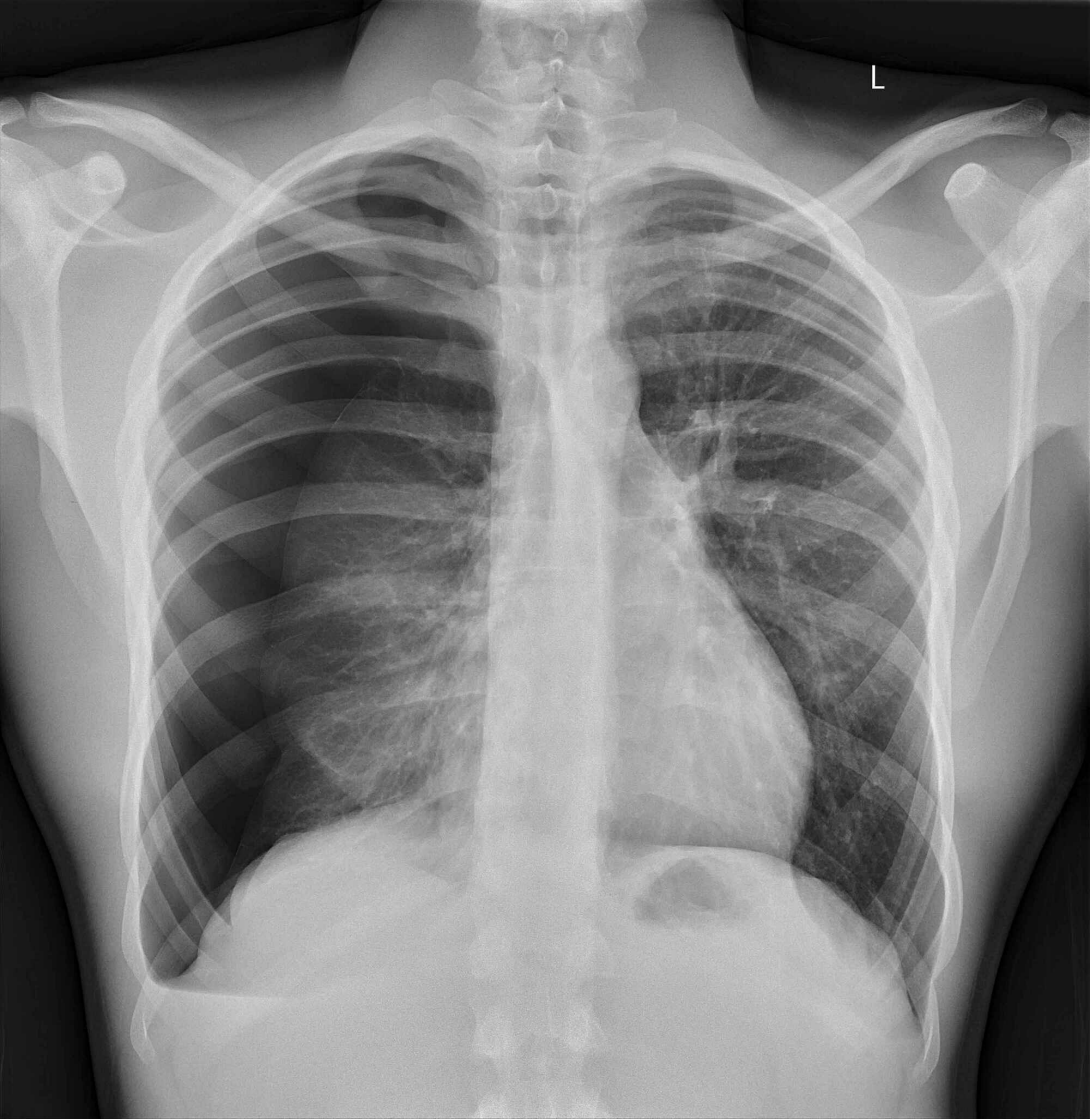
Chest x-ray showing tension pneumothorax Case courtesy of Dr. Ana Brusic, Radiopaedia.org, rID: 60551.
